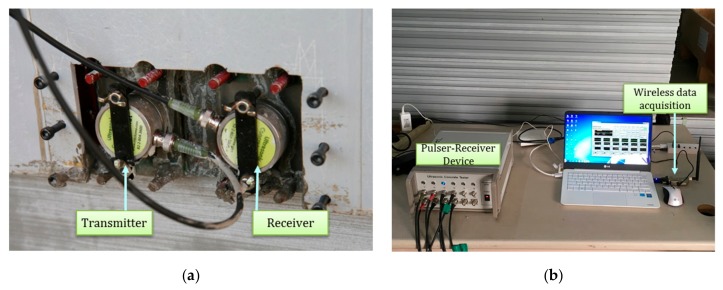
Measurement setup for slip-forming: (a) ultrasonic transducers, (b) data acquisition system [5]; the models of the ultrasonic transducer, the pulser-receiver, and the wireless data acquisition unit are CT-1010, Ultracon-3030, and MKDQ-710 of MKC Korea, respectively.
